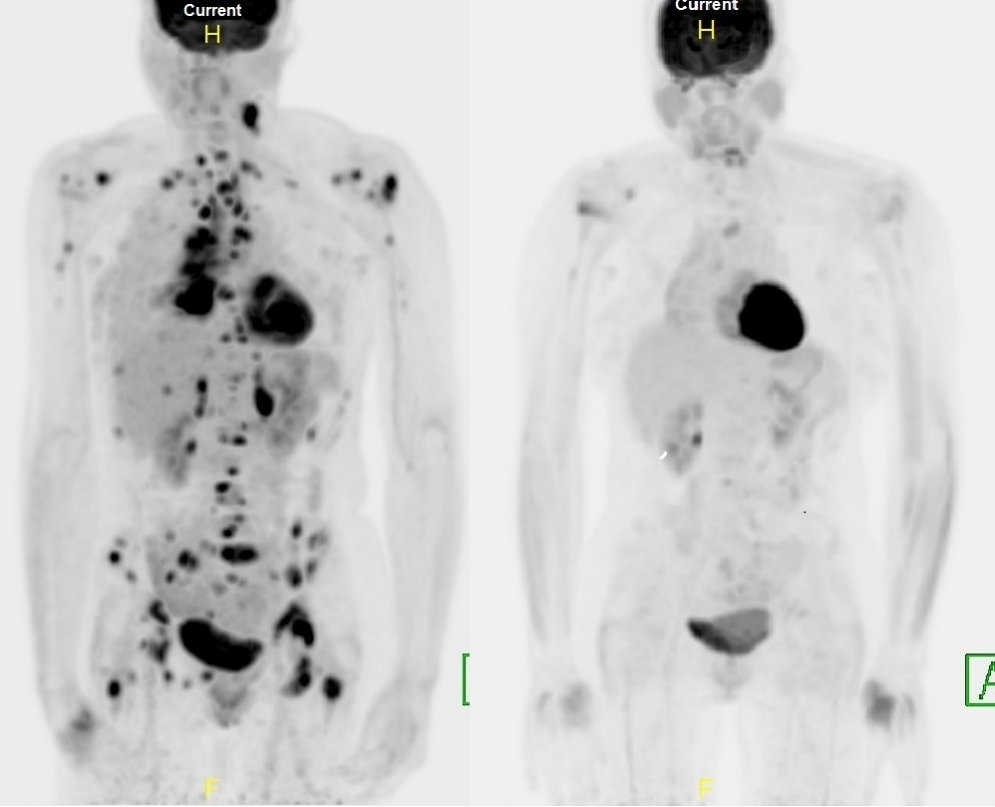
Baseline and four-month positron emission tomography-computed tomography (PET-CT) scans
